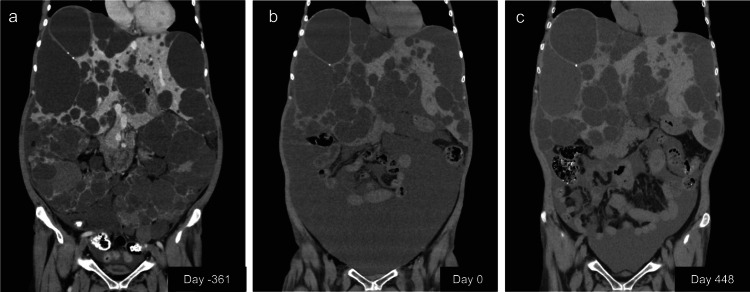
Coronal abdominal computed tomography (CT) findings. a Preoperative (day -361) CT image demonstrating markedly enlarged polycystic kidneys and multiple hepatic cysts, resulting in severe abdominal distension. b CT image obtained at admission (day 0), showing massive ascites. c CT image obtained on day 448, 140 days after the second CART session, showing a reduction in ascites
